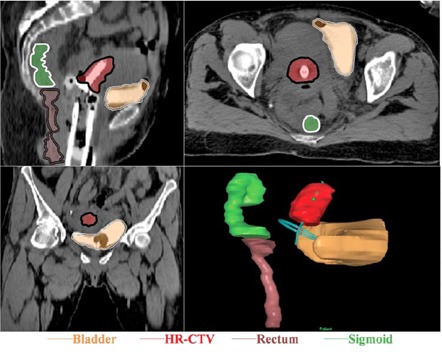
CT image data for the delineated regions of interest as displayed in the planning system and the three‐dimensional view of the contours.
